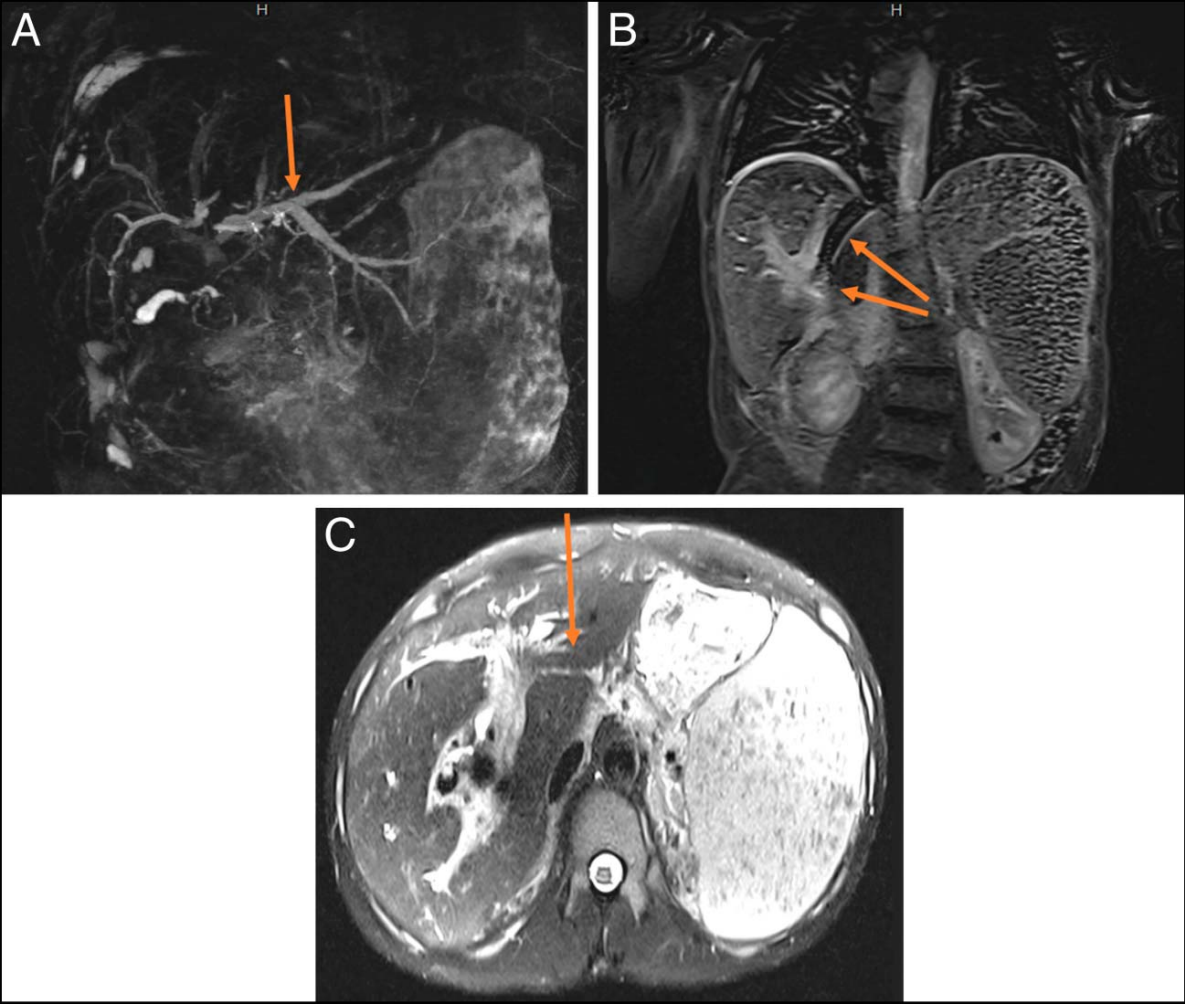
Magnetic resonance imaging of the abdomen and pelvis (T2 haste) showing a column of air within the TIPS stent and fistulous connection between the TIPS and CBD stents in different views: (a) intrahepatic biliary ductal dilation (MRCP 3D reconstruction), (b) the TIPS stent with intraluminal air (upper arrow) and concern for fistulous connection with the CBD stent (lower arrow) (axial view), and (c) the TIPS stent with intraluminal air (coronal view). CBD, common bile duct; MRCP, magnetic resonance cholangiopancreatography; TIPS, transjugular intrahepatic portosystemic shunt.
